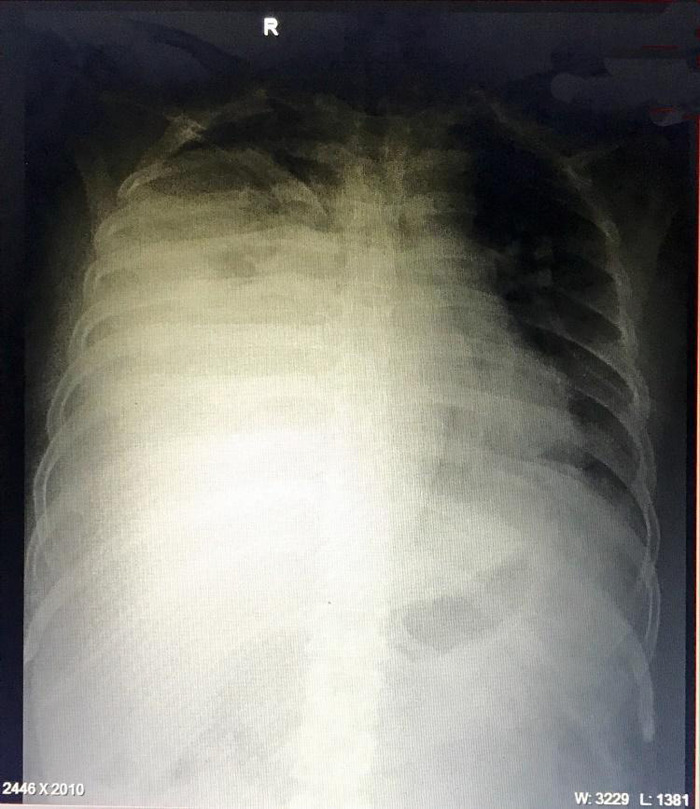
X-ray showing opacity of the right lung.
